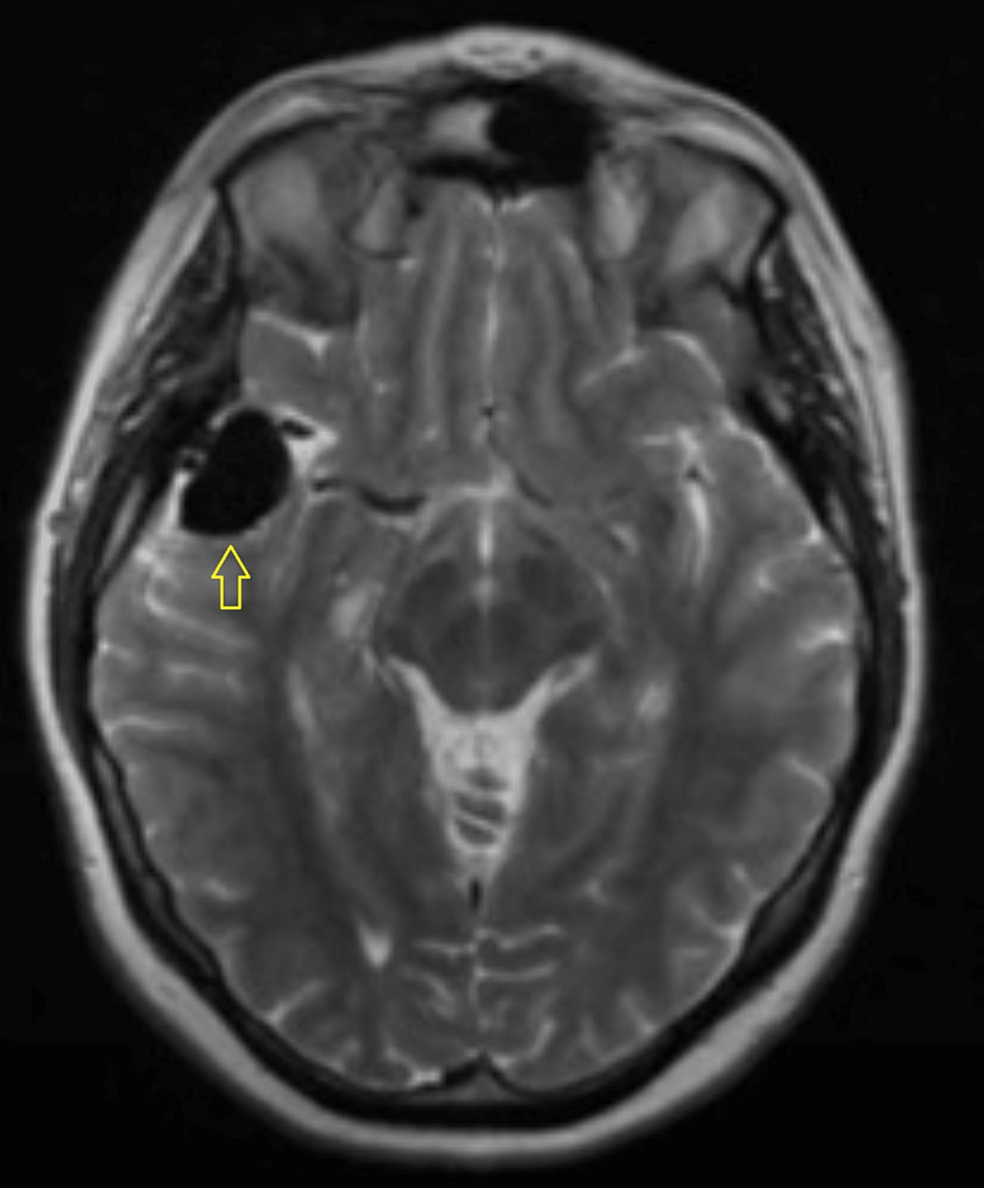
MRI scan showing dilated venous varix in the right Sylvian fissure
